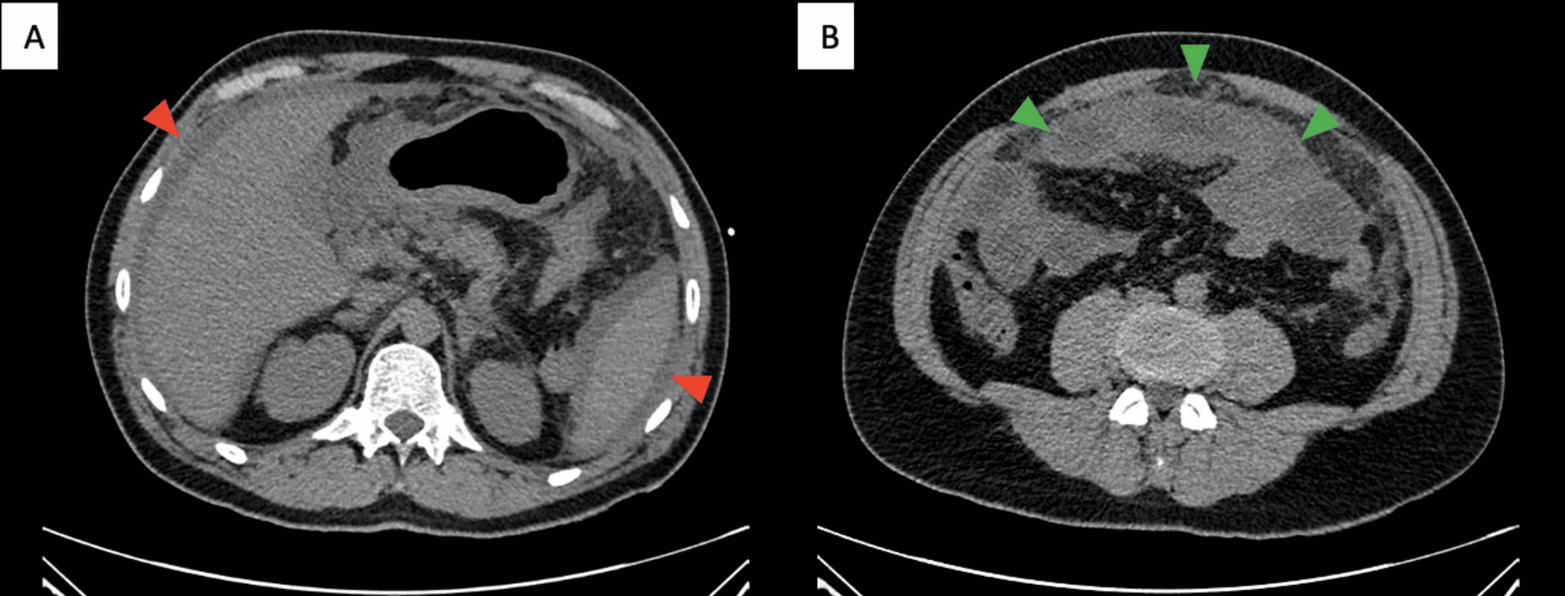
Computed tomography in spontaneous contrast on the day of admission Two images showing axial scans through the upper third (A) and middle third (B) of the abdomen. It shows diffuse perihepatic and perisplenic ascites (red arrows), diffuse mesenteric infiltration, and dilated small intestine loops (green arrows).
